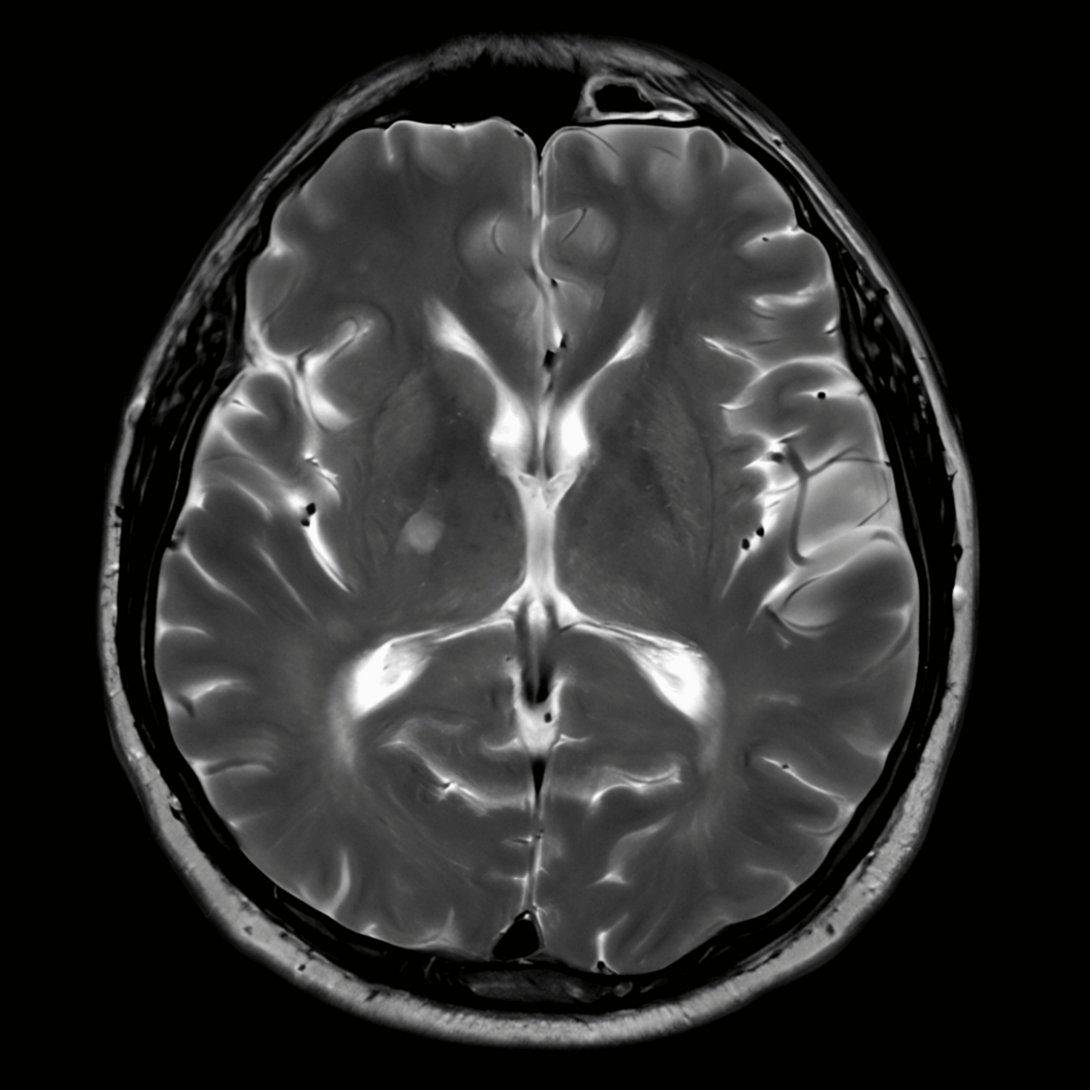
MRI head The image showed T2/FLAIR hyperintensities in the bilateral periventricular and deep white matter, with a small area of restricted diffusion adjacent to the posterior limb of the right internal capsule, consistent with a small area of ischemic infarction MRI: magnetic resonance imaging; FLAIR: fluid-attenuated inversion recovery
